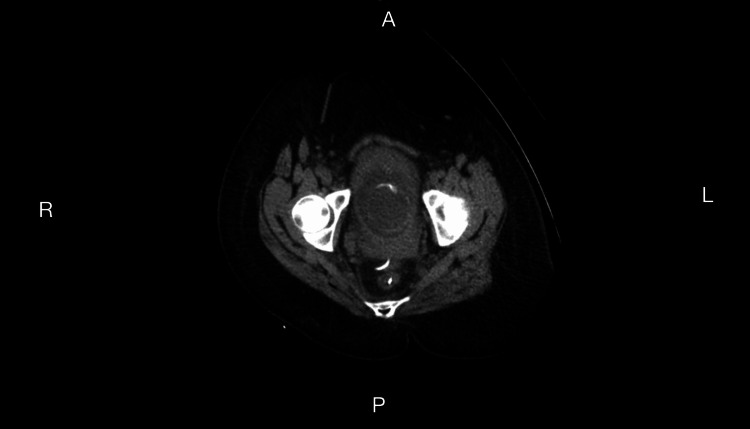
Drain Visualized in Abscess Impression: CT-guided right 8 French transgluteal drain placement into rectouterine pouch fluid collection.
